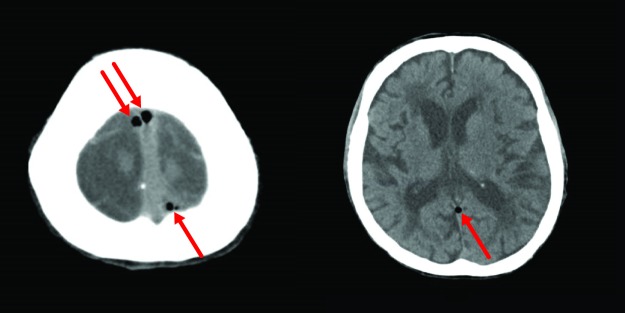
Initial CT Brain Initial computed tomography (CT) brain showing air within the superior sagittal (left) and straight sinuses (right)
